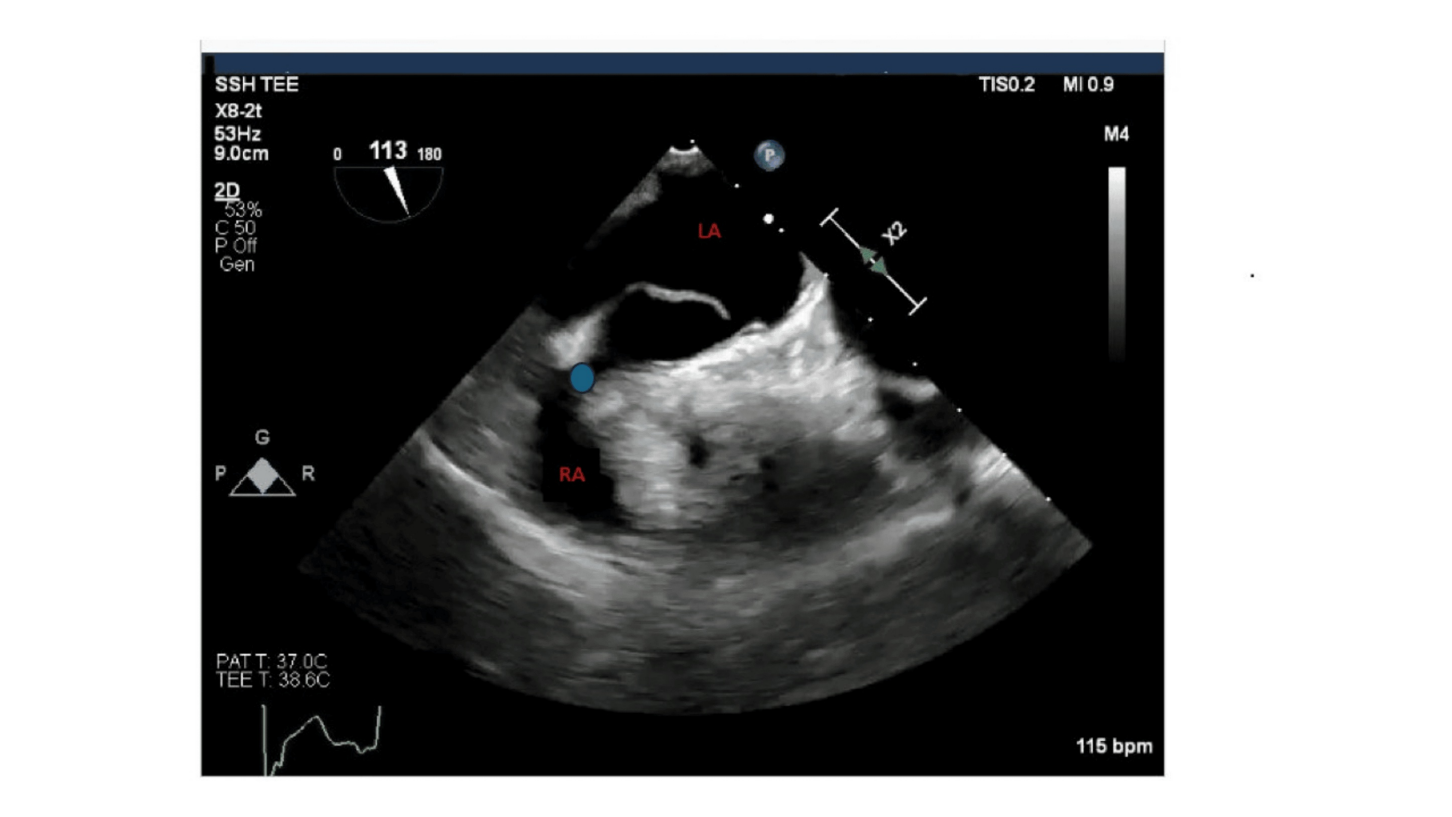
TEE bicaval view depicting PFO (blue circle). The blue circle draws focus to the conduit between the left and right atria or the patent foramen ovale. The photo was captured during a trans-esophageal echocardiogram where a scope was inserted into the patient's mouth and down the esophagus to acquire views of the heart's chambers. The bicaval view of this echocardiogram allows visualization of the left atrium, right atrium, inter-atrial septum, superior vena cava, and inferior vena cava. TEE: Transesophageal Echocardiogram; PFO: Patent Foramen Ovale; RA: Right Atrium; LA: Left Atrium
